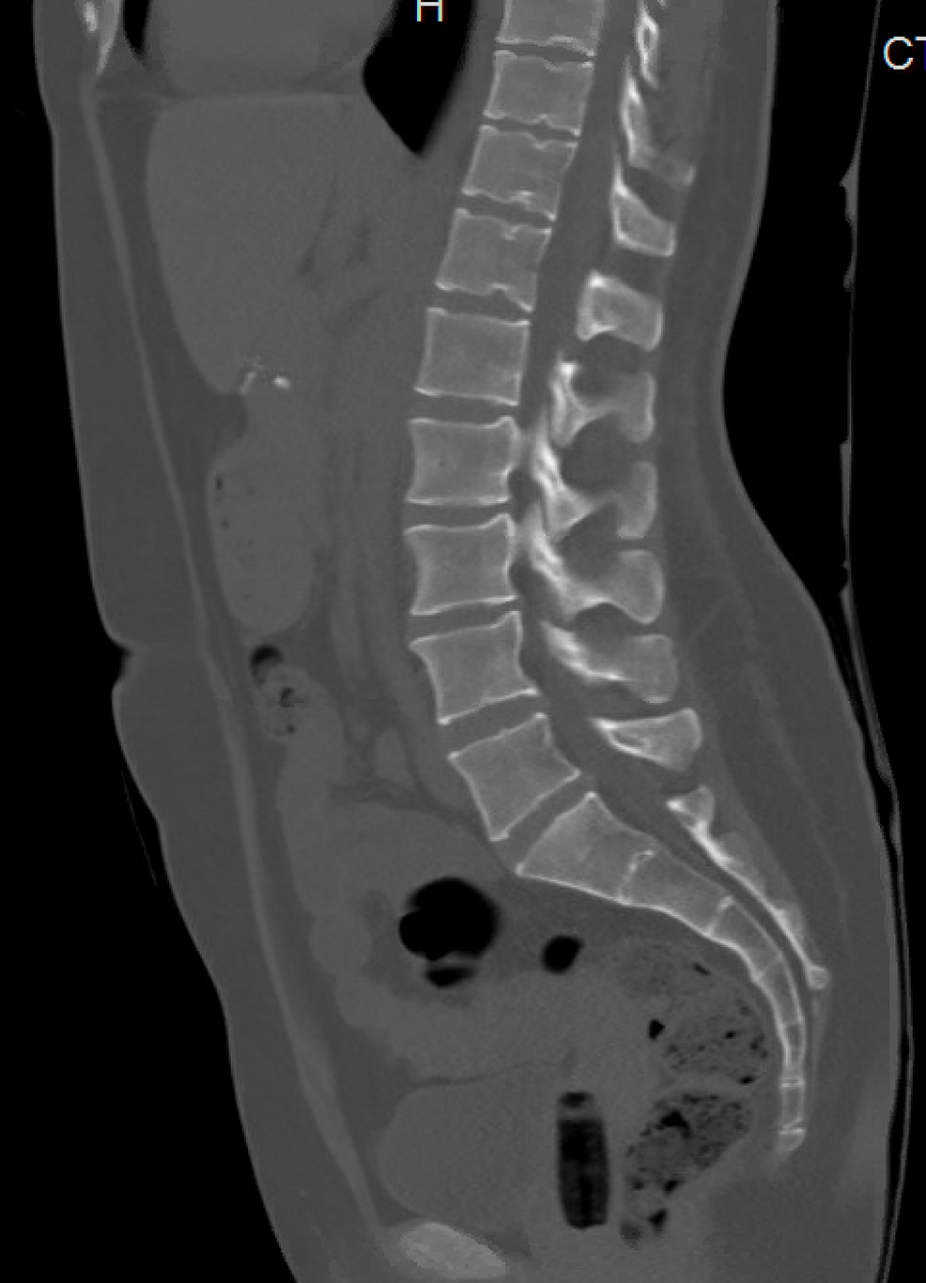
CT of the lumbar spine not suggestive of osteolytic lesions
